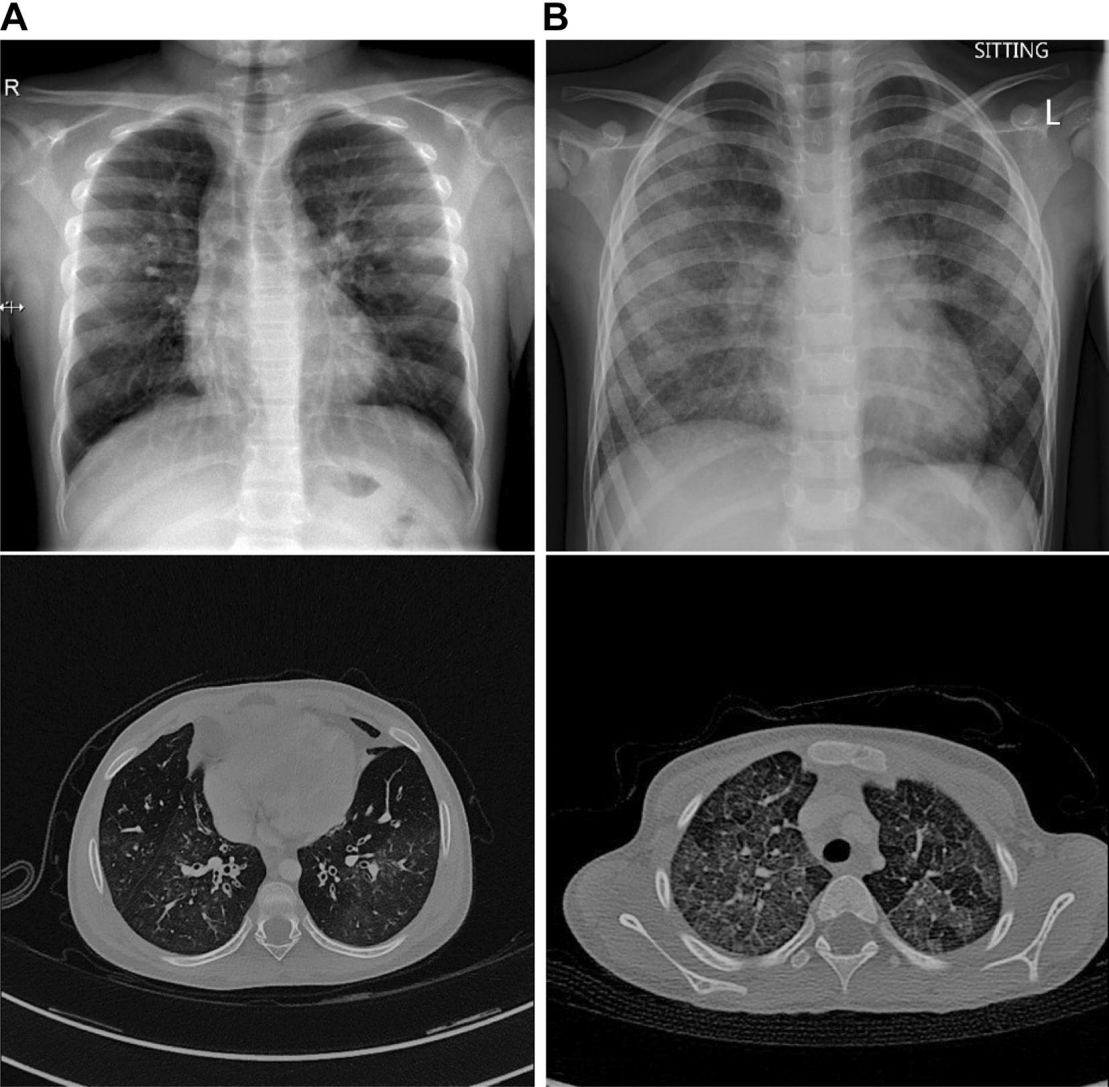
(A) Chest radiograph and unenhanced high resolution chest CT axial image of an 18-month-old girl with heterozygous *SCNN1B*:p.Arg206Trp. The chest radiograph shows hyperinflated lungs with bronchial wall thickening and band of atelectasis. The chest CT image demonstrates features of air-trapping, bronchial wall thickening and mild bronchiectasis. (B) Chest radiograph and unenhanced high resolution chest CT axial image of an 18-month-old girl with heterozygous *SFTPA1*:p.Gly98Ala. The chest radiograph shows bilateral and quite symmetrical ground-glass opacification, relatively spares the lung apices. The chest CT image demonstrates a combination of septal thickening and alveolar ground-glass opacification creates a typical pattern of crazy-paving.
